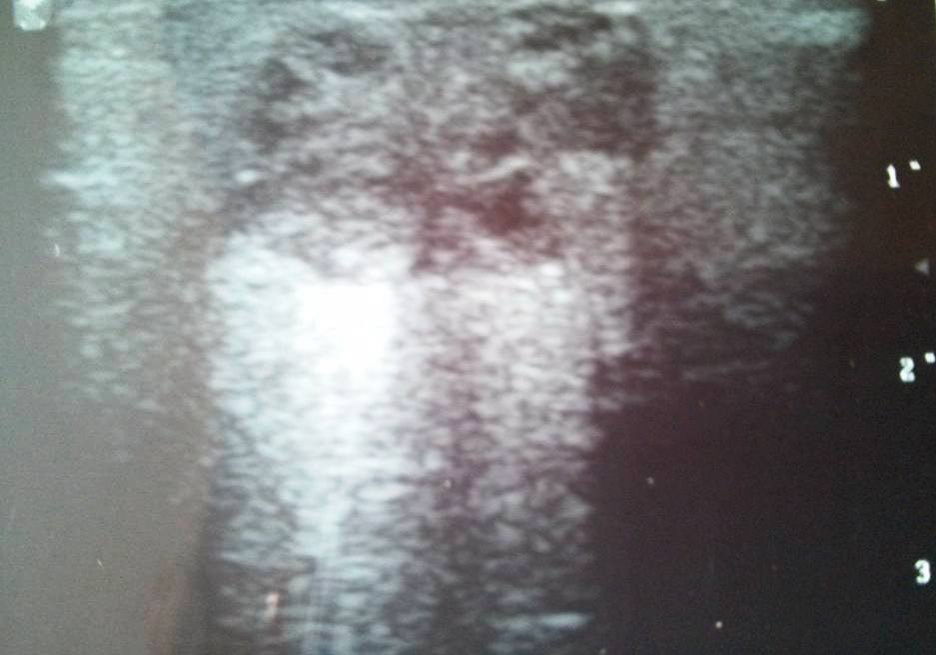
Radiograph reveals calcifications of the parotid space
